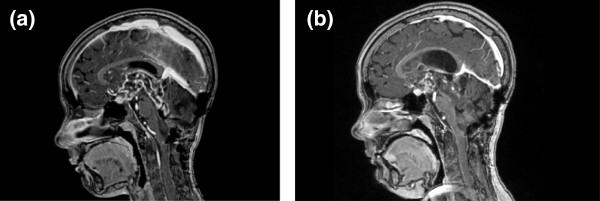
Cerebral MRI, sagittal sections, T1 weighted with gadolinium injection. (a) May 2009: Before antitumoral treatment with celecoxib/fluvastatin. Hypersignal in the hypothalamo-chiasmatic region, fourth ventricle, cerebellum. Post-operative reshuffle of the posterior fossa. (b) September 2010: After 16 months of antitumoral treatment with celecoxib/fluvastatin. Decrease in contrast enhancement of the hypothalamo-chiasmatic, fourth ventricle and cerebellum lesions.
